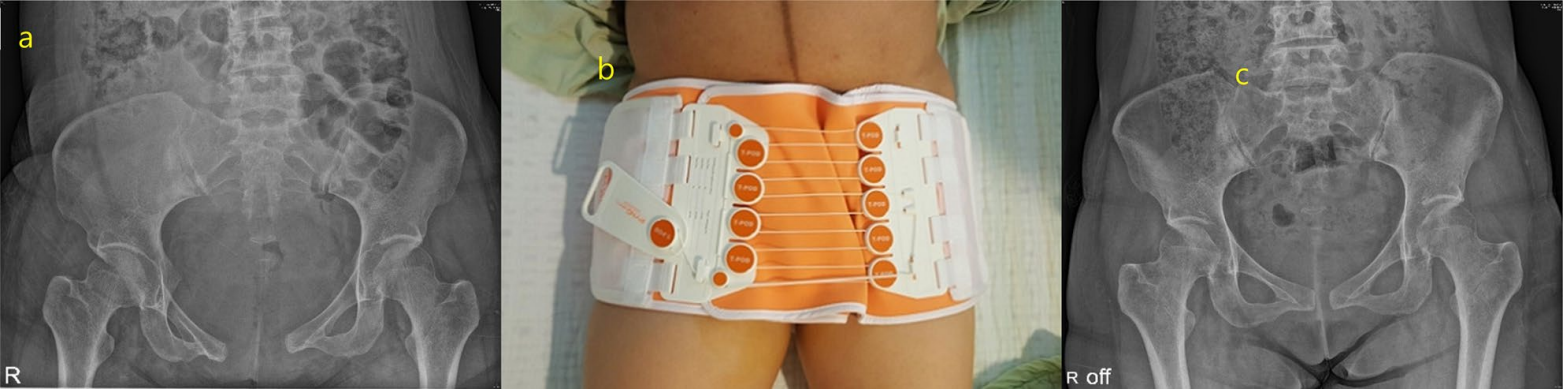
A 34-year-old woman who had pubic pain and gait difficulty after vaginal delivery was diagnosed as PPSD. (a) Pelvic radiography at postpartum day 1 showing 5 cm separation of the pubic symphysis. (b) She was applied pelvic binder to support pelvic ring integrity. (c) Pelvic radiograph at 2-month follow-up showing decreased separation of 1.15 cm.
